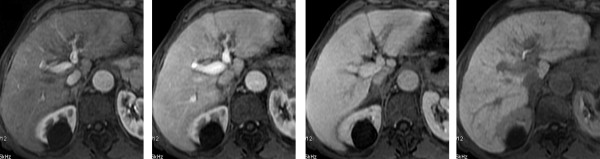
Twelve-months follow up with MRI before and after after injection of Primovist: 5a, T1-weighted; 5b, T2-weighted:On baseline T1w.i and T2w.i no sign of changes of signal intensity within the liver parenchyma is appreciable, not even at the level of the previous lesion of segment III.
